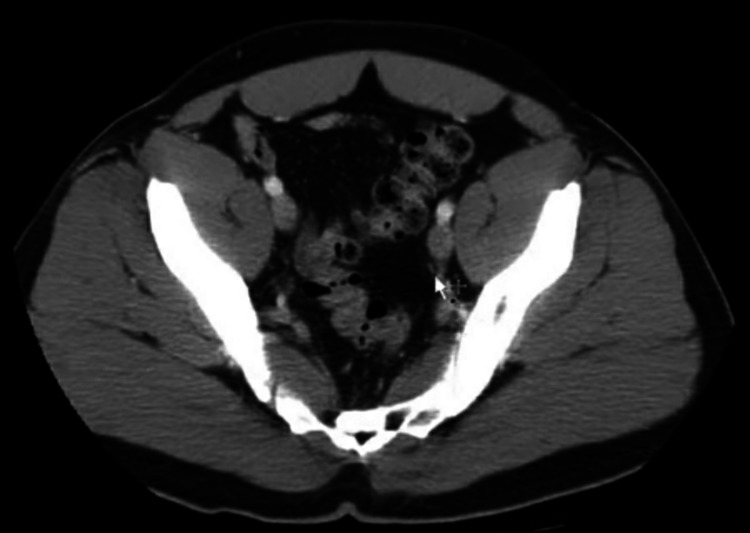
CT scan of the abdomen and pelvis 10mm axial section through the abdomen and pelvis with oral and non-ionic low osmolar IV contrast: white arrow showing no adjacent metastasis.
